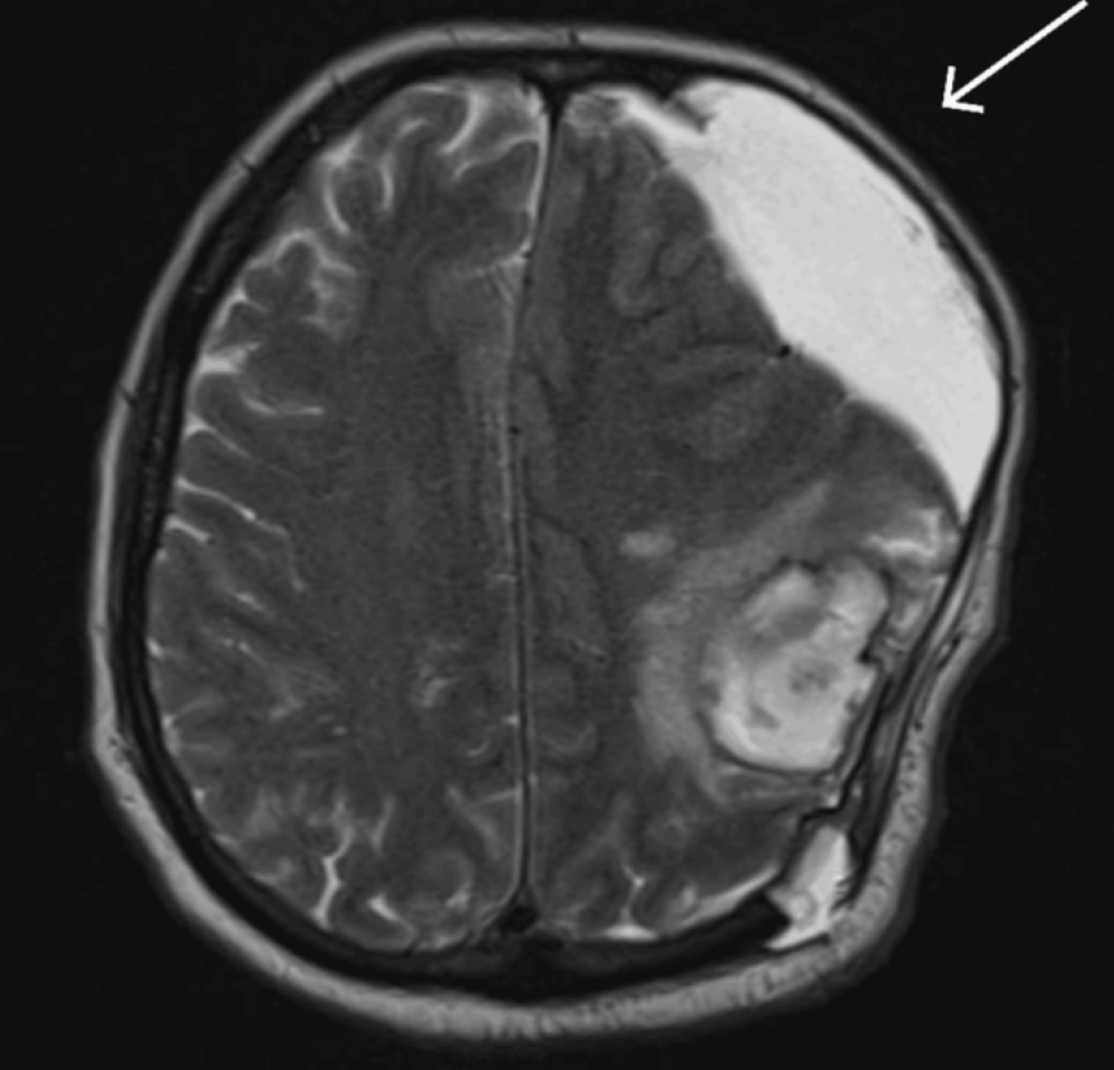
MRI T2-weighted images showing post-op changes, with the arrow pointing towards the hematoma.
